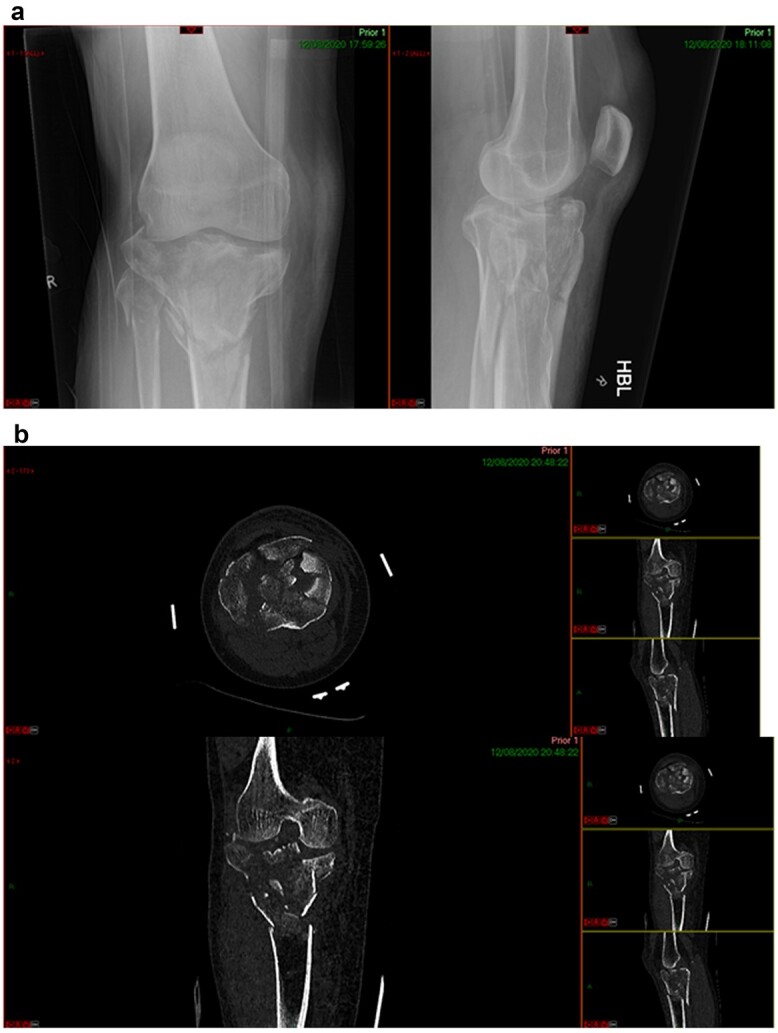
(a) Post-injury radiograph, (b) post-injury CT scan.
